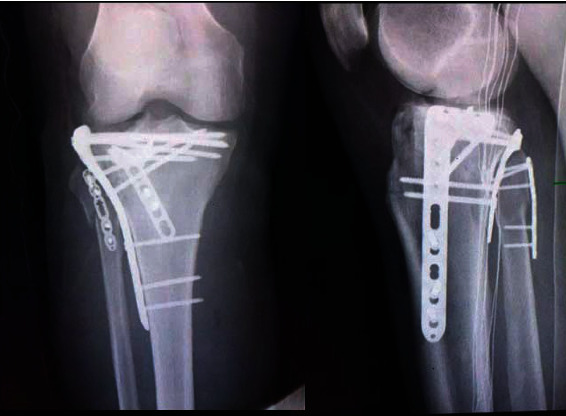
A type 2 case fixed by a mini locking plate: (a, b) preoperative X-ray.
